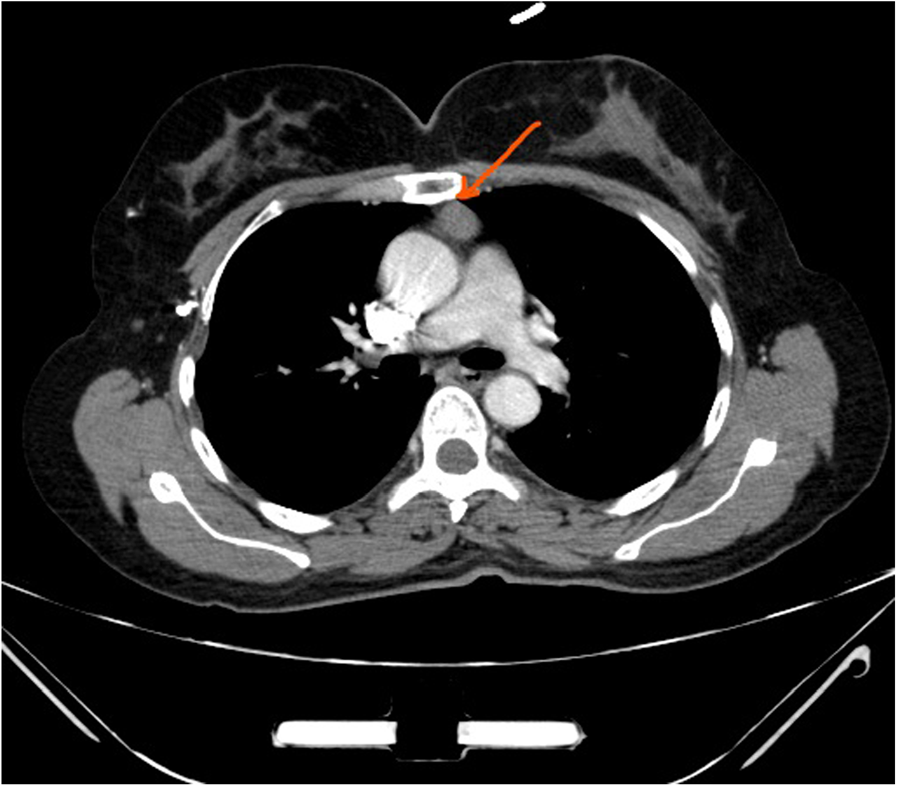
CT scan of the chest showing a 2 × 2 cm well-defined oval shaped enhancing soft tissue anterior mediastinal tumor with (Hounsfield units of 55)
